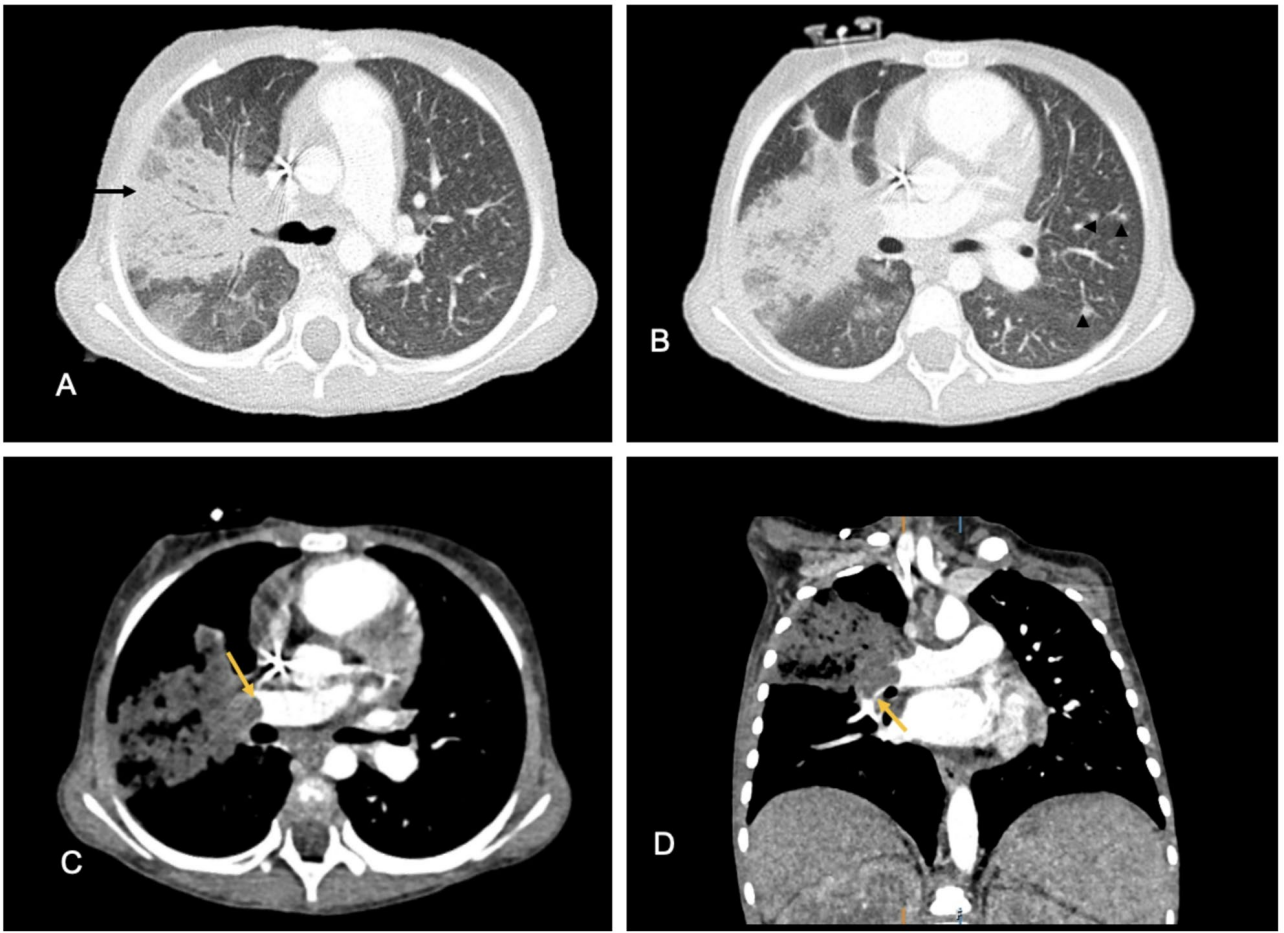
Disseminated candida infection in a 3-year-old girl with relapsed B-cell acute lymphoblastic leukemia at 10 months after CAR T-cell therapy. Imaging performed 10 months post–CAR T-cell infusion during an episode of febrile neutropenia and persistent Candida fungemia demonstrates: A) Axial chest CT shows patchy, multisegmented air-space consolidation with positive bronchograms in the right upper lobe, consistent with a pneumonia (arrow). B) Axial chest CT shows additional centrilobular nodules in the left lower love (arrowheads). C) Axial contrast enhanced chest CT reveals invasion of the pulmonary infiltrate into the main right pulmonary artery (arrow). D) Coronal contrast enhanced chest CT reveals invasion of the pulmonary infiltrate into the right lower lobe arteries (arrow)
